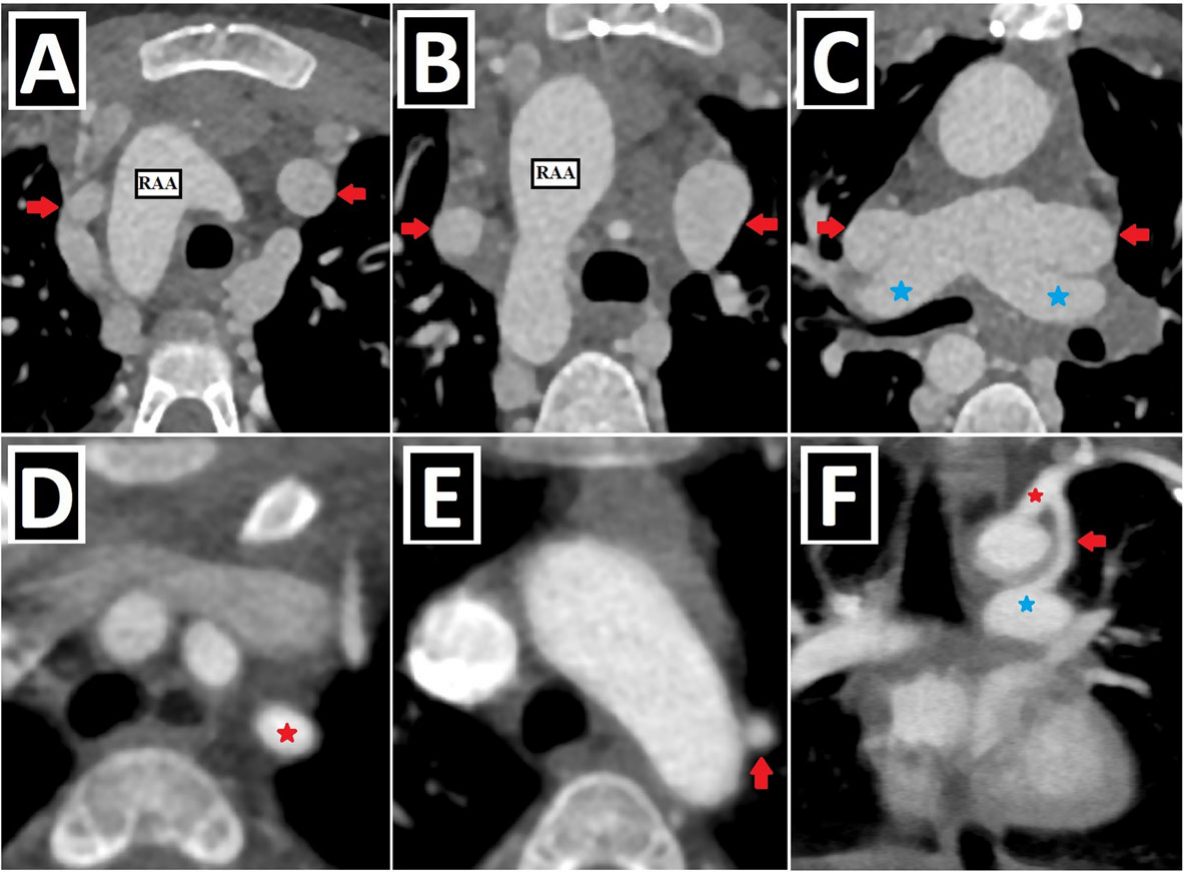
Vascular structures secondary to surgery. a–c Axial CT images of the patient with the right aortic arch (RAA) and complex cardiac anomaly depict bicaval Glenn shunt (red arrows), which is an anastomosis constructed between the right and left SVCs and right and left pulmonary arteries (blue stars, c), respectively. After the performing surgical connection between the PLSVC and the left pulmonary artery, the PLSVC draining to the left pulmonary artery is called the Glenn shunt. e–f Axial CT images of the patient with the TOF show BT shunt (red arrows) connecting the left subclavian artery (red stars) and the left pulmonary artery (blue star). The distinction of PLSVC from the BT shunt is quite easy by establishing that the vascular structure in the left mediastinum (e) originates from the left subclavian artery and ends in the left pulmonary artery (f)
